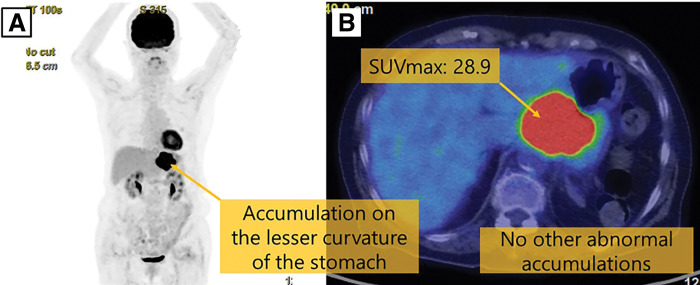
PET-CT scan images. (A, B) PET-CT scan revealed accumulation of SUV_max_ 28.9 in the tumor, but no other abnormal accumulation was noted.
